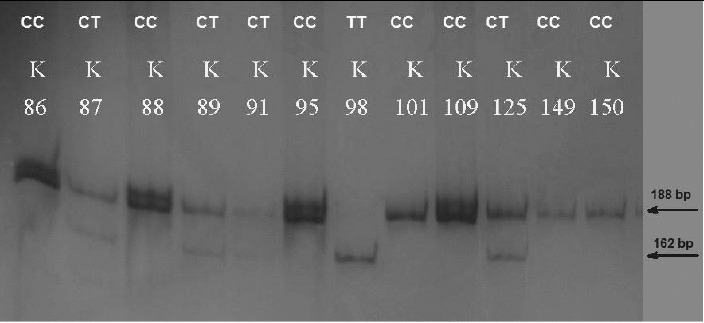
PAGE for visualization of PCR-RFLP products and genotyping for *MMP2* −*1306C>T*. The homozygous *CC* carriers were determined with one visible band of 188 bp (K86, K88, K95, K101, K109, K149, K150); the heterozygous *CT* carriers with two visible bands of 188 bp and 162 bp (K87, K89, K91, K125) and the homozygous *TT* carriers with one visible band of 162 bp (K98).
